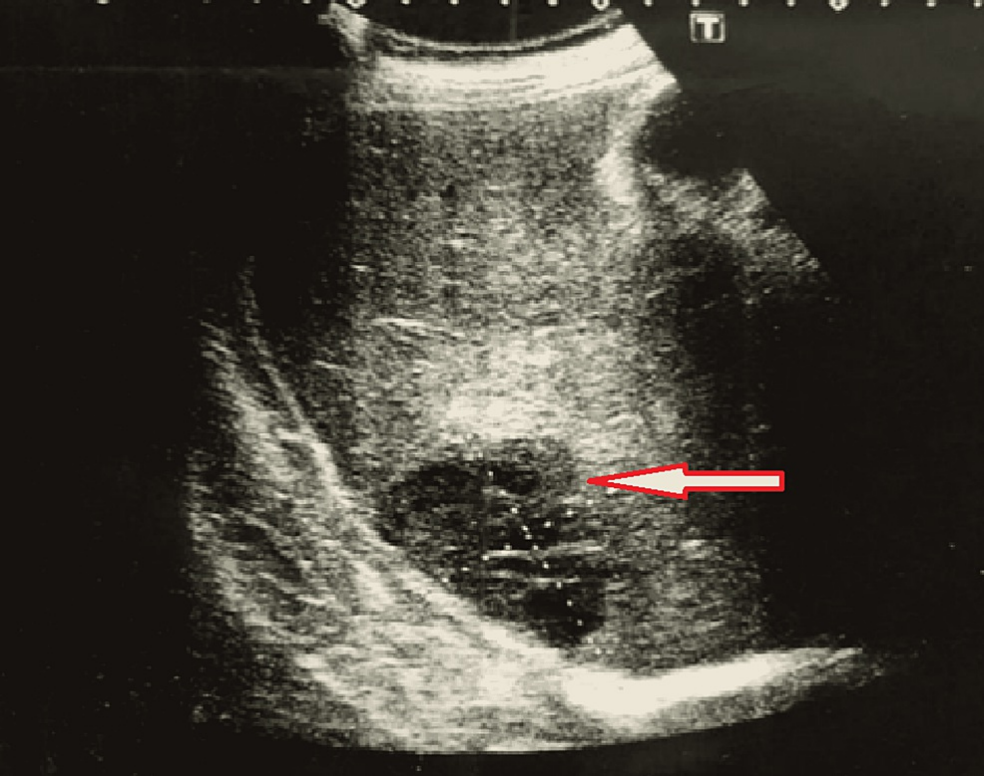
Ultrasound of the abdomen revealing hepatic abscess Arrow indicates a hypoechoic solitary lesion measuring 4.6 × 5.7 cm in size in the subdiaphragmatic region of the right lobe of the liver (segment vii) favoring liver abscess.
